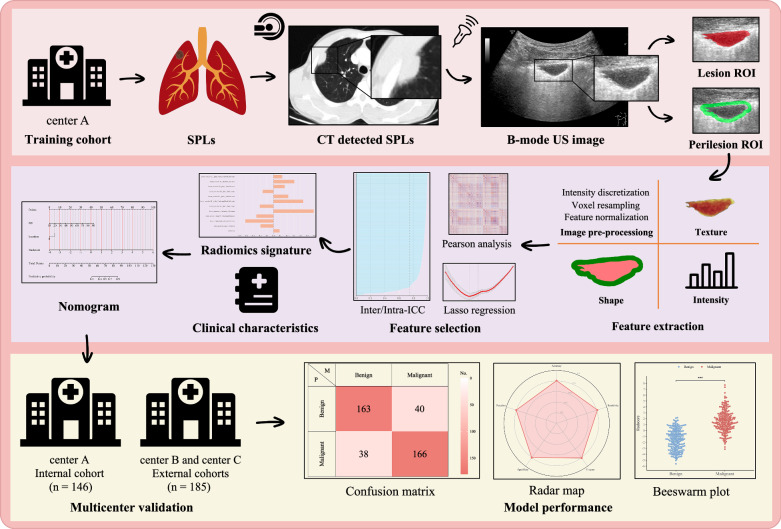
Workflow of the study design and radiomics analysis. SPLs, subpleural pulmonary lesions; CT, computed tomography; US, ultrasound; ROI, region of interest; Lasso, least absolute shrinkage and selection operator; ICC, inter-/intra-class correlation coefficients
